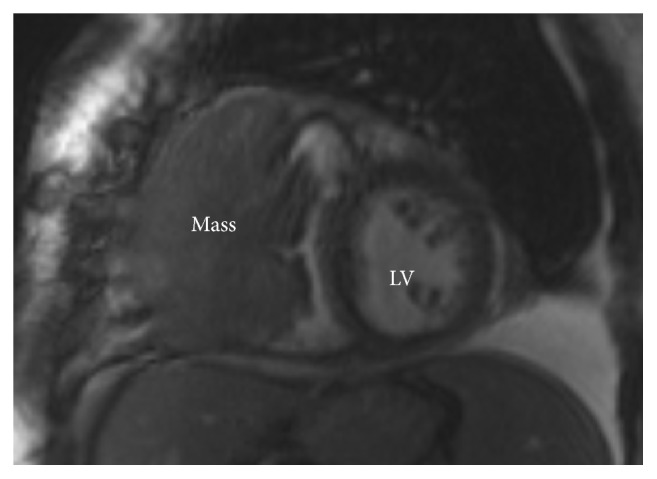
Cardiac MRI image showing a parasternal short axis view at the level of the papillary muscles demonstrates a large mass in the RV.
